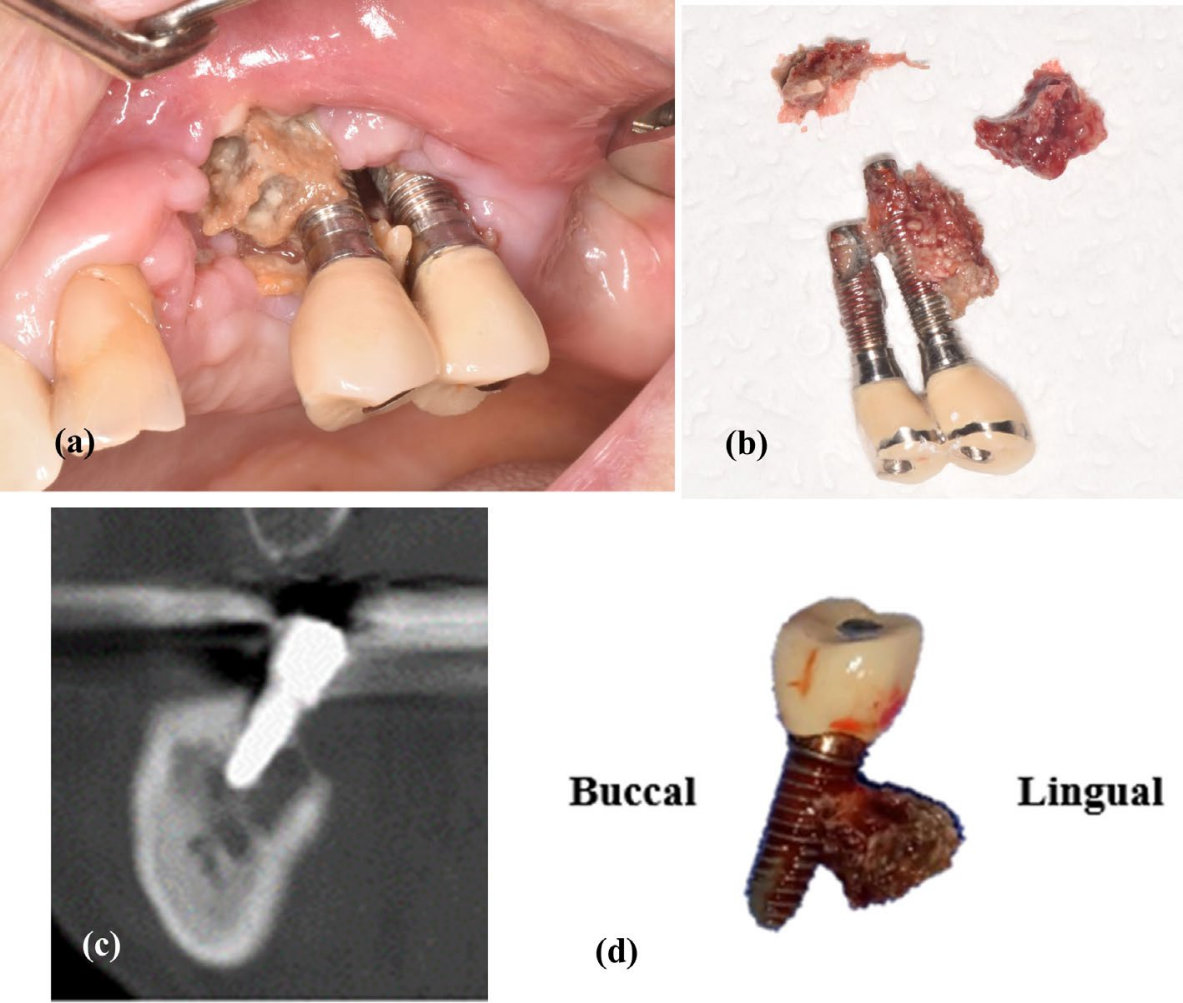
Clinical aspect of the bone necrosis areas affecting the implant region. (a, b) Representative clinical photographs of peri‐implant medication‐related osteonecrosis of the jaw. (c) Preoperative radiographic view with lingual tilted inclination. (d) Postoperative image showing implant fixture with a sequestrum.
